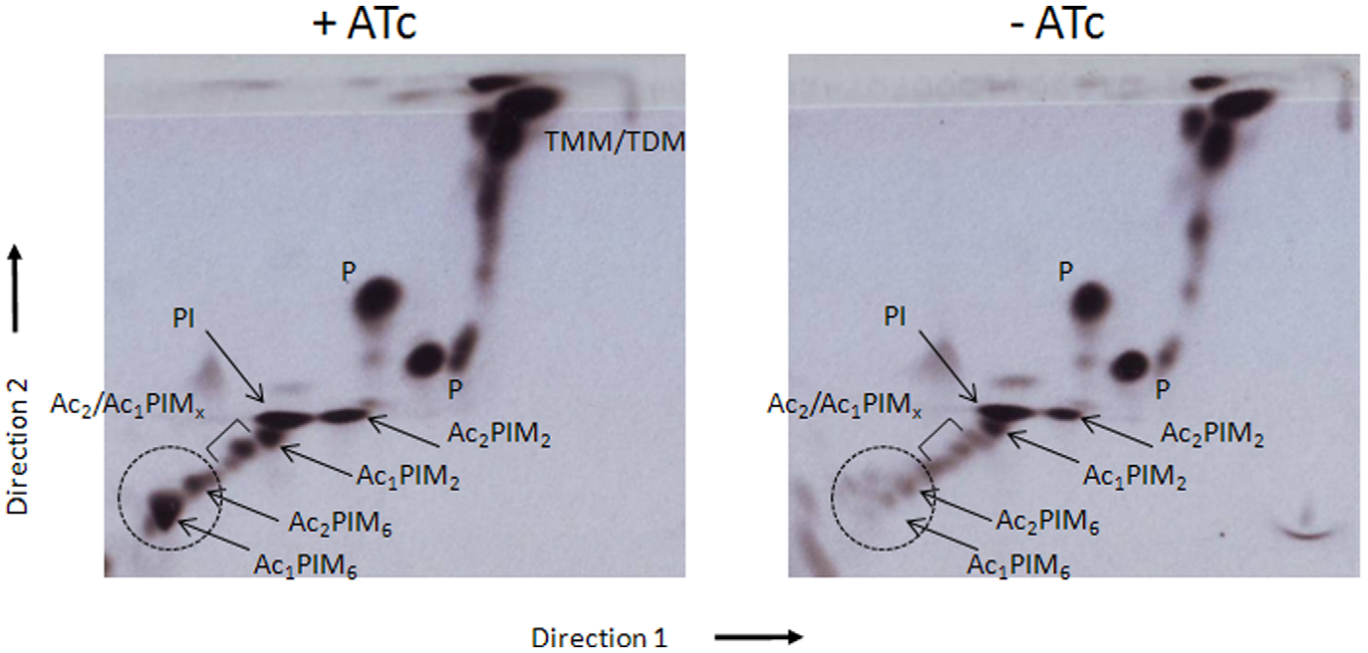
2D-TLC analysis of [^14^C]-labeled polar lipids from the ΔMsPpm conditional mutant. Cultures were grown and labelled in Tryptic Soy Broth in the presence or the absence of anhydrotetracycline (ATc). Equal counts of polar lipid extracts (50 000 cpm) were applied to Silica Gel 60 F_254_ (Merck 5554) aluminium-backed TLC plates and developed using solvent system E for polar lipids: CHCl_3_/CH_3_OH/H_2_O (60∶30∶6, v/v/v) in the first direction and CHCl_3_:CH_3_OOH: CH_3_OH:H_2_O (40∶25∶3∶6, v/v/v/v) in the second direction. Polar lipids were visualized by 48 h exposure on x-ray films by autoradiography (Kodak Biomax MR film). PIM intermediates are shown by arrows (x = 3–5) and the dotted circle indicates the position of the higher PIMs on the TLC plates.
